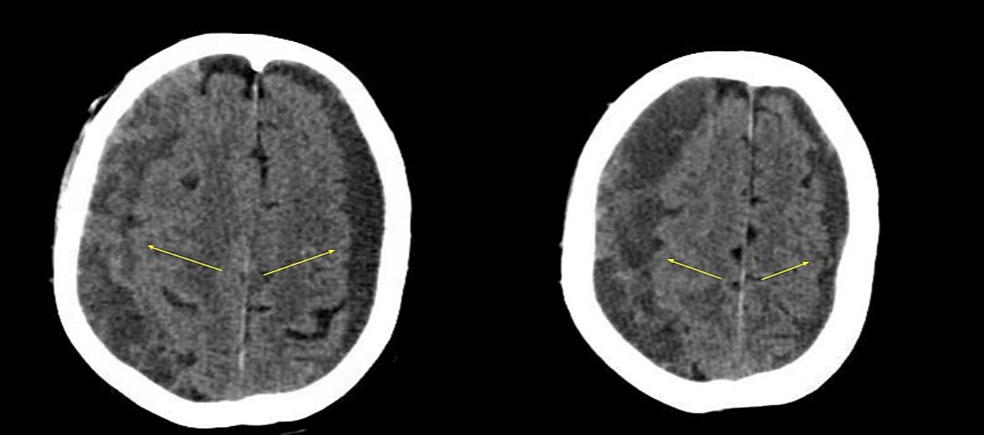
Head axial computed tomographic scan showing a bilateral chronic subdural hematoma (yellow arrows).
